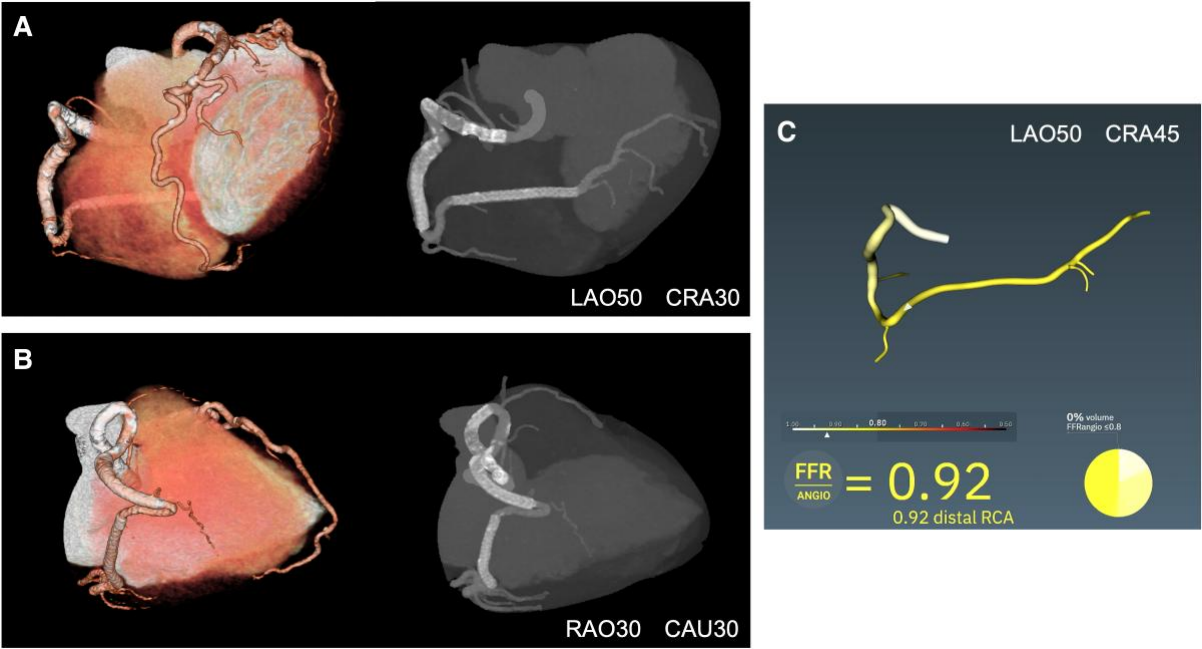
Follow-up computed tomography and angiography-based fractional flow reserve. (*A* and *B*) Follow-up computed tomography imaging at 6 months demonstrated excellent coronary artery patency. The mildly curved segments were treated with drug-eluting stents, and severely tortuous segments were treated with drug coated balloons. (*C*) Angiography-based fractional flow reserve revealed substantial coronary blood flow at 12 months after percutaneous coronary intervention.
